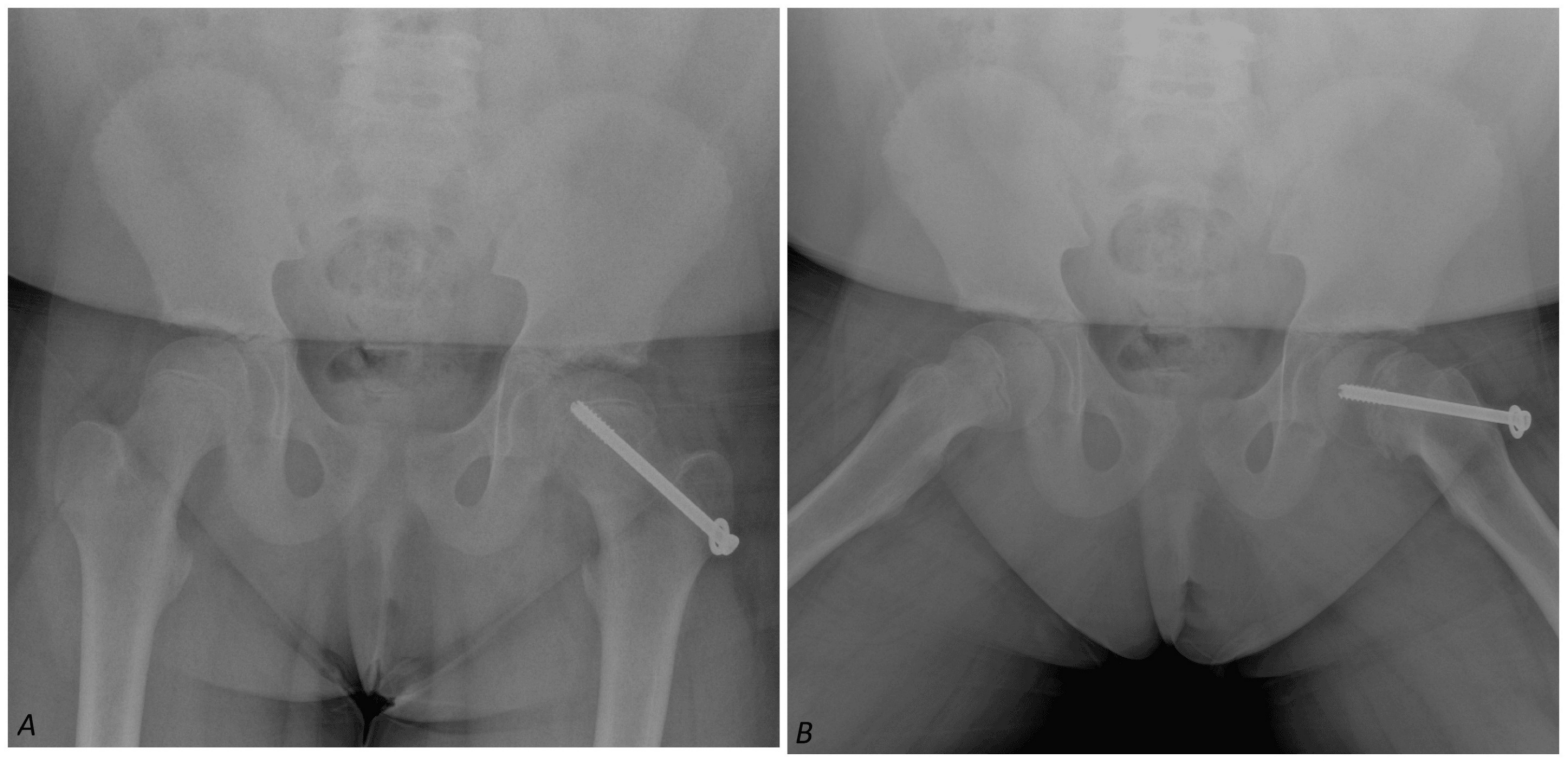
AP (A) and frog leg (B) X-ray views, showing pin fixation of SUFE in left hip without any evidence of SUFE in the right hip. SUFE: Slipped Upper Femoral Epiphysis, AP: Anteroposterior
